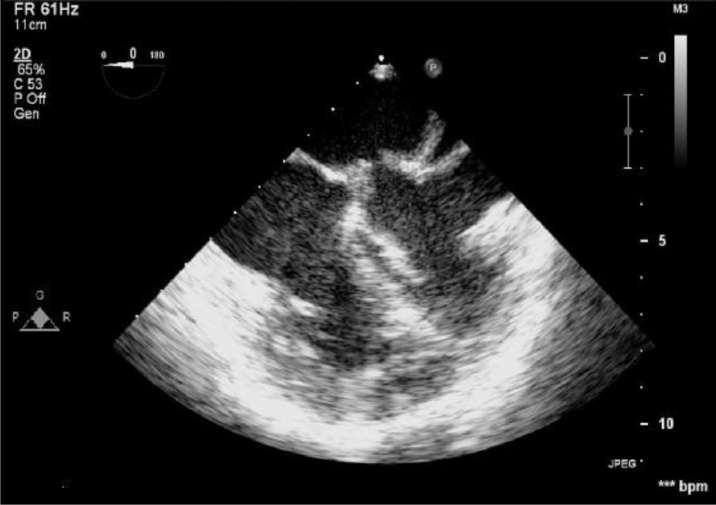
Transesophageal Echocardiography Image Four-chamber view shows the fibromuscular membrane causing septation of the left atrium.
